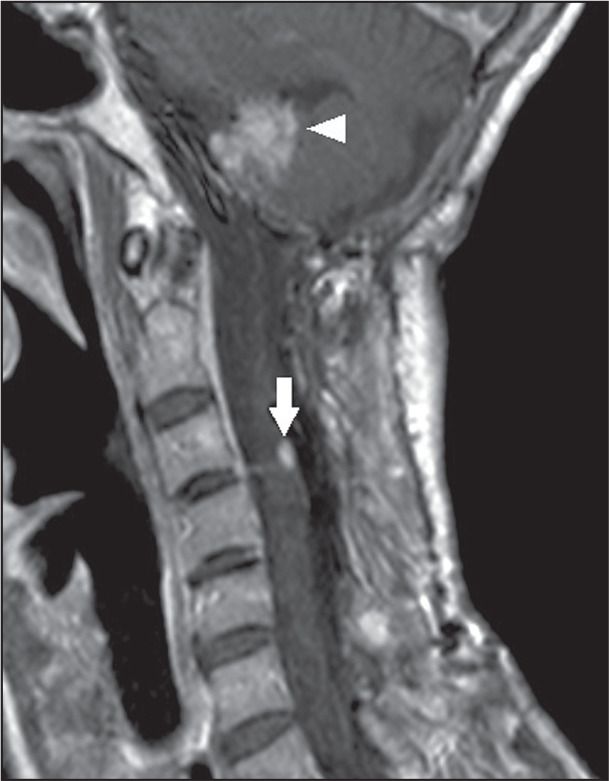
Hemangioblastoma. Sagittal MRI scan of a patient with Von Hippel-Lindau
